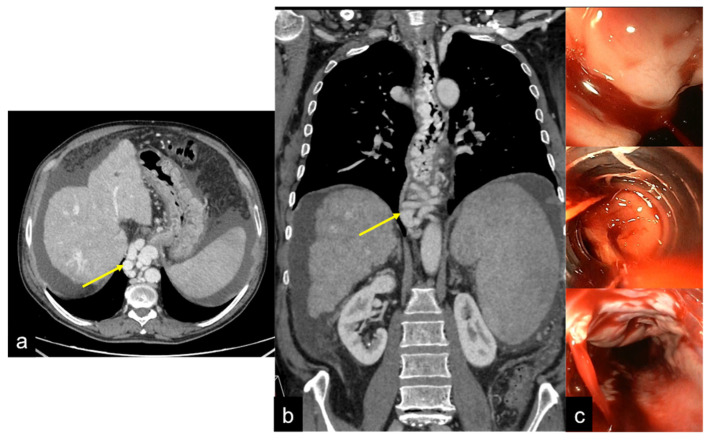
Axial (a) and coronal (b) images of CTA multiphasic study with endoscopic corresponding images (c). In CT, the oesophageal varices in portal hypertension are visualised as tubular structures with an endoluminal protrusion in the oesophageal wall ((a,b) arrow); the endoscopic evaluation reveals signs of active bleeding (c).
